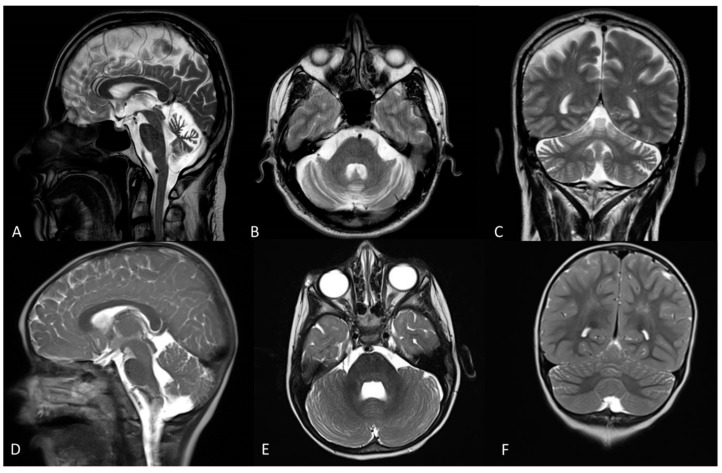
Brain MRI of patient 1 performed at 57 years old. T1-weighted midsagittal (A) axial (B) and coronal (C) sections showing atrophy of the cerebellar vermis and hemispheres. Brain MRI of patient 2 performed at age 2 years. T1-weighted midsagittal (D) axial (E) and coronal (F) sections showing mild atrophy of the superior cerebellar vermis and hemispheres.
